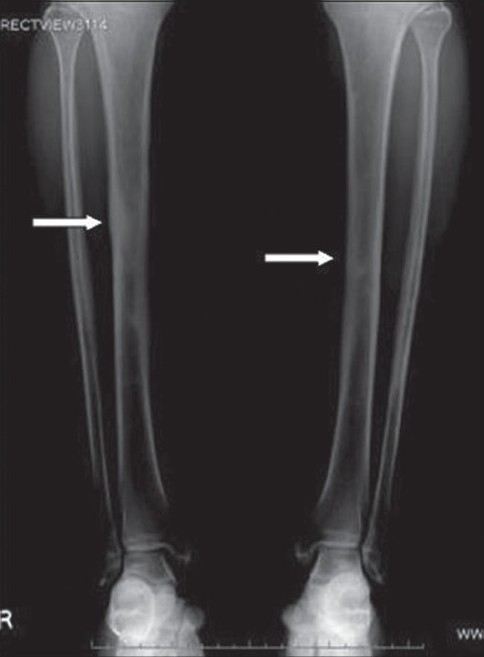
Frontal radiograph of both legs reveals sclerosis of the mid diaphysis of both tibias, right more than left (arrows)
